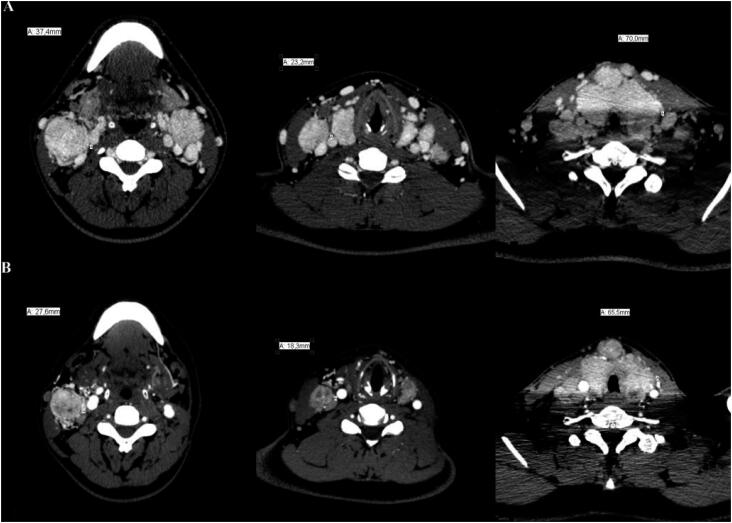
(A) Initial CT scans revealing thyroid enlargement with tracheal stenosis and bilateral conglomerates of cervical lymph nodes with compression of adjacent structures. (B) CT scans after 12 months of sorafenib therapy, demonstrating significant reduction of neck disease.
